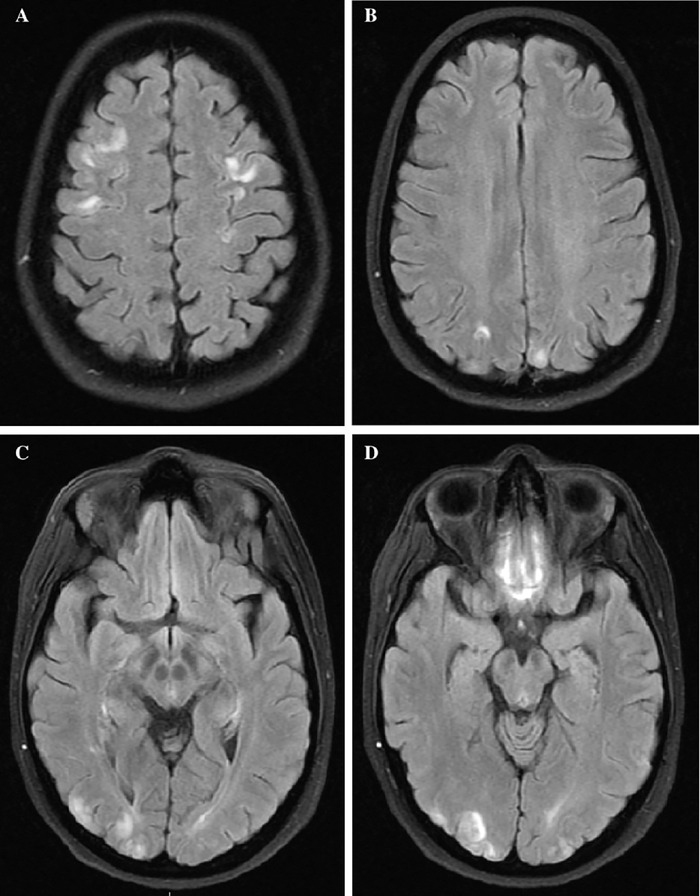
Magnetic resonance imaging axial T2-weighted fluid-attenuated inversion recovery with fat suppression images show multiple cortical and subcortical hyperintensities in the (A) bilateral parietal lobes, (B) parieto-occipital region, (C) bilateral occipital lobes, and (D) bilateral frontal lobes suggestive of posterior reversible encephalopathy syndrome.
